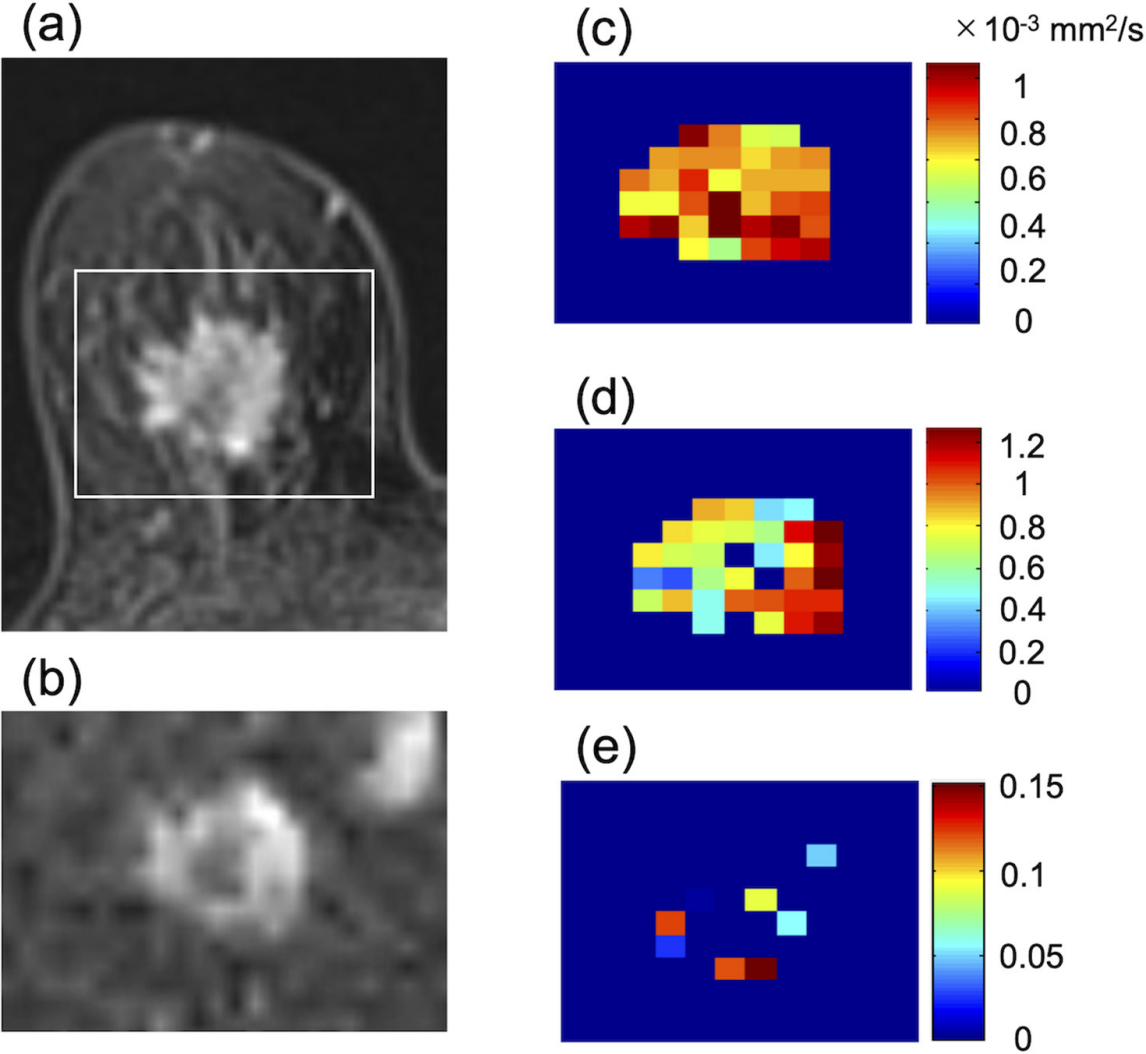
Images of a 77-year-old female with invasive ductal carcinoma who developed pleural metastases 5 years and 11 months after MR examination. (a) contrast-enhanced axial MR image, (b) DWI (*b* = 1500 s/mm^2^), (c) ADC_0_ map, (d) K map, (e) fIVIM map. The white rectangle on (a) shows the area covered by the parametric maps. (a) The mass lesion is heterogeneously enhancing. (b) The lesion shows heterogeneously high signal intensity on DWI. (c) ADC_0_ map shows low values throughout the lesion with a mean value of 0.9 × 10–3 mm^2^/s. (d) The mass shows relatively high mean K values (1.0) with heterogeneous distribution. (e) fIVIM map shows low values throughout the lesion with a mean value of<0.01. (Reprint from Ref. 27).
